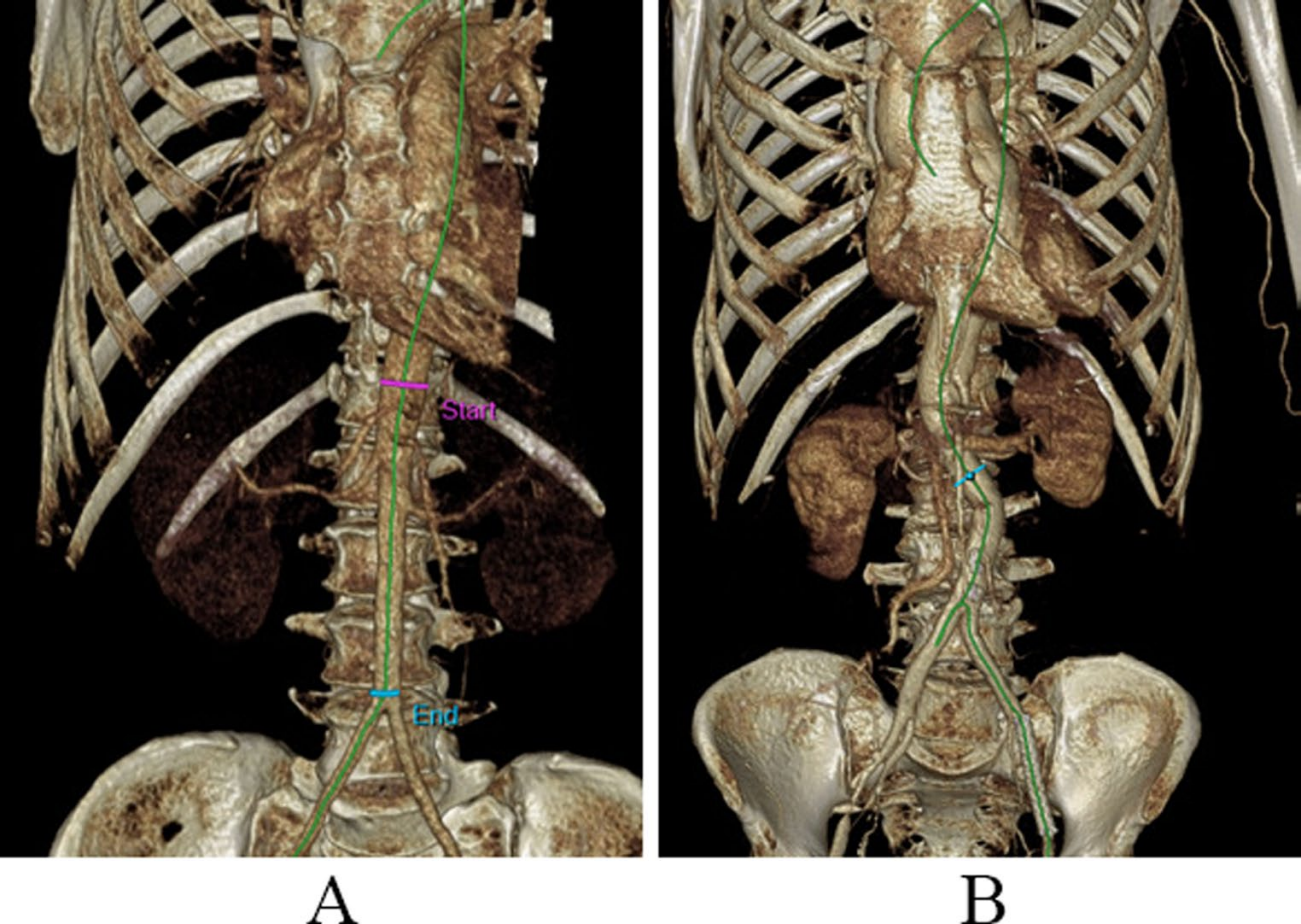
Computed tomography angiography scans showing straight (a) and C – shaped (b) tortuosity of the abdominal aorta
